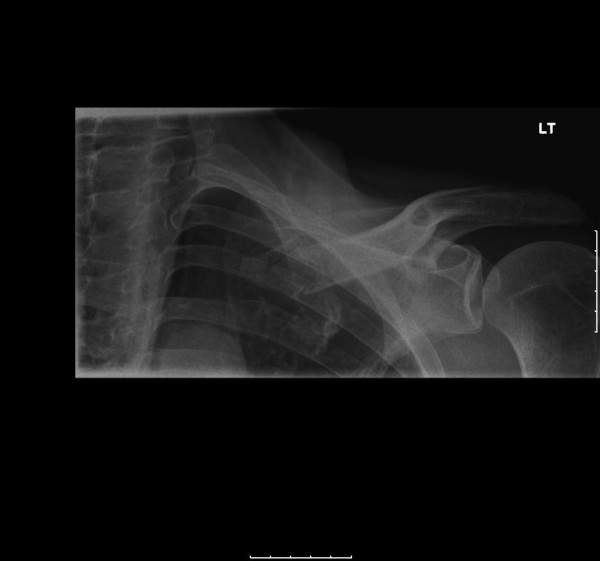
AP radiograph of the left clavicle demonstrating a fracture of the medial third with an opacity extending from the fracture site in the soft tissues of the neck (day 8).
